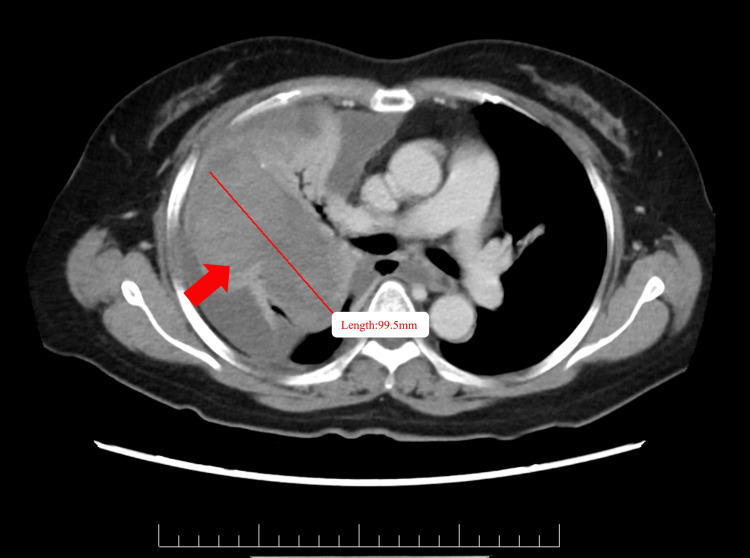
Chest CT imaging. The CT scan demonstrates a pulmonary mass in the right lower lobe, as indicated by the red arrow. The red line delineates the lesion’s maximum diameter, highlighting its extent.
